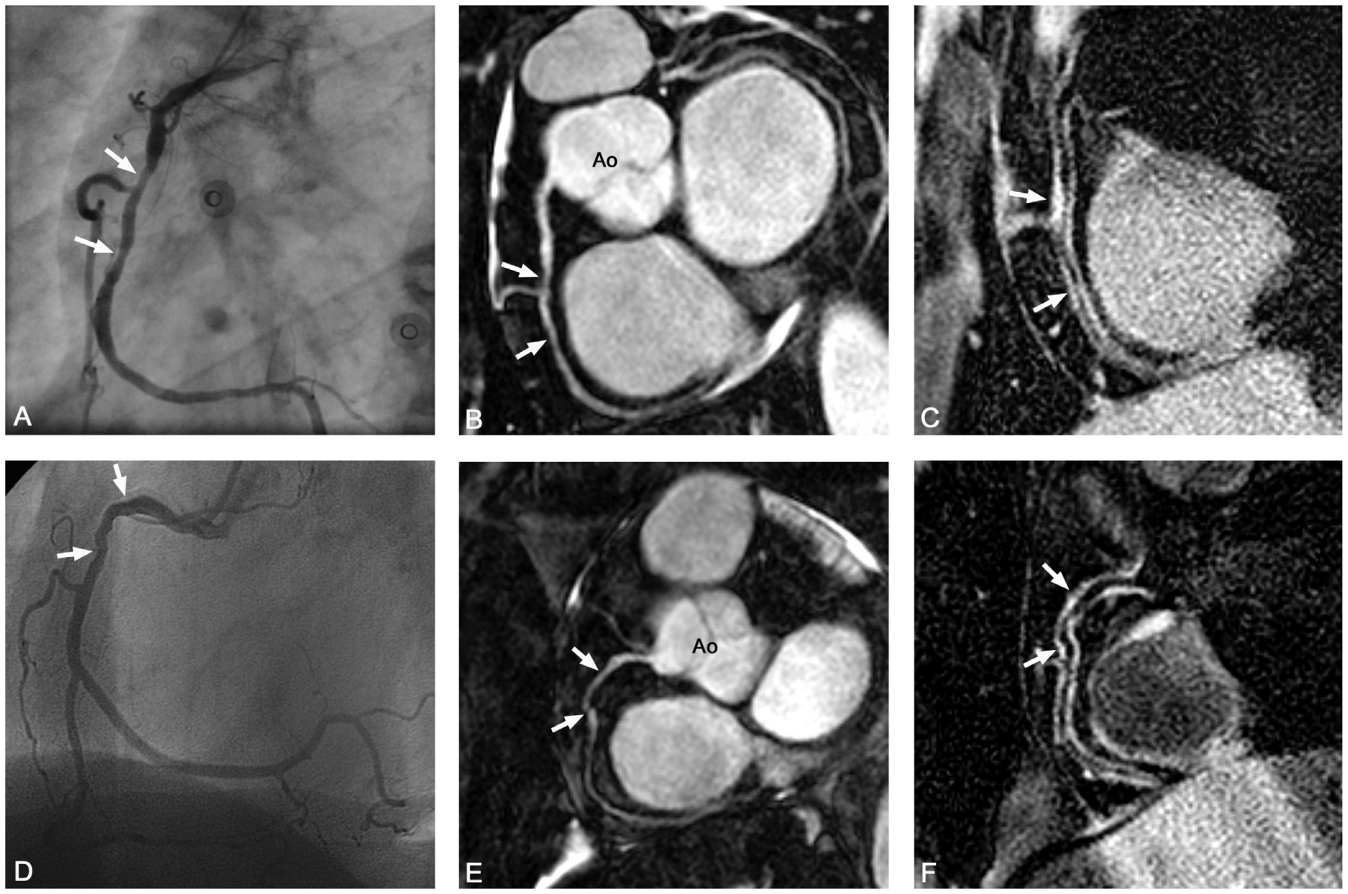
MRA and MR-CVW in patients with stable angina. X-ray coronary angiography (A,D), balanced steady state free precession (bSSFP) coronary MRA (B,E) and corresponding vessel wall scans (C,F) of the right coronary artery (RCA) in two patients with stable angina. In a 40 y/o male (A–C), vessel wall thickness and signal intensity are increased in areas of stenosis and wall irregularities (arrows). In a 67 y/o female (D–F) the culprit lesion was located in the left anterior descending artery (not imaged). However, the proximal RCA demonstrated wall focal luminal irregularities (arrows in D,E). In F, corresponding focal heterogeneity in wall thickness and signal intensity of the entire vessel wall are clearly visible. Ao indicates aorta.
